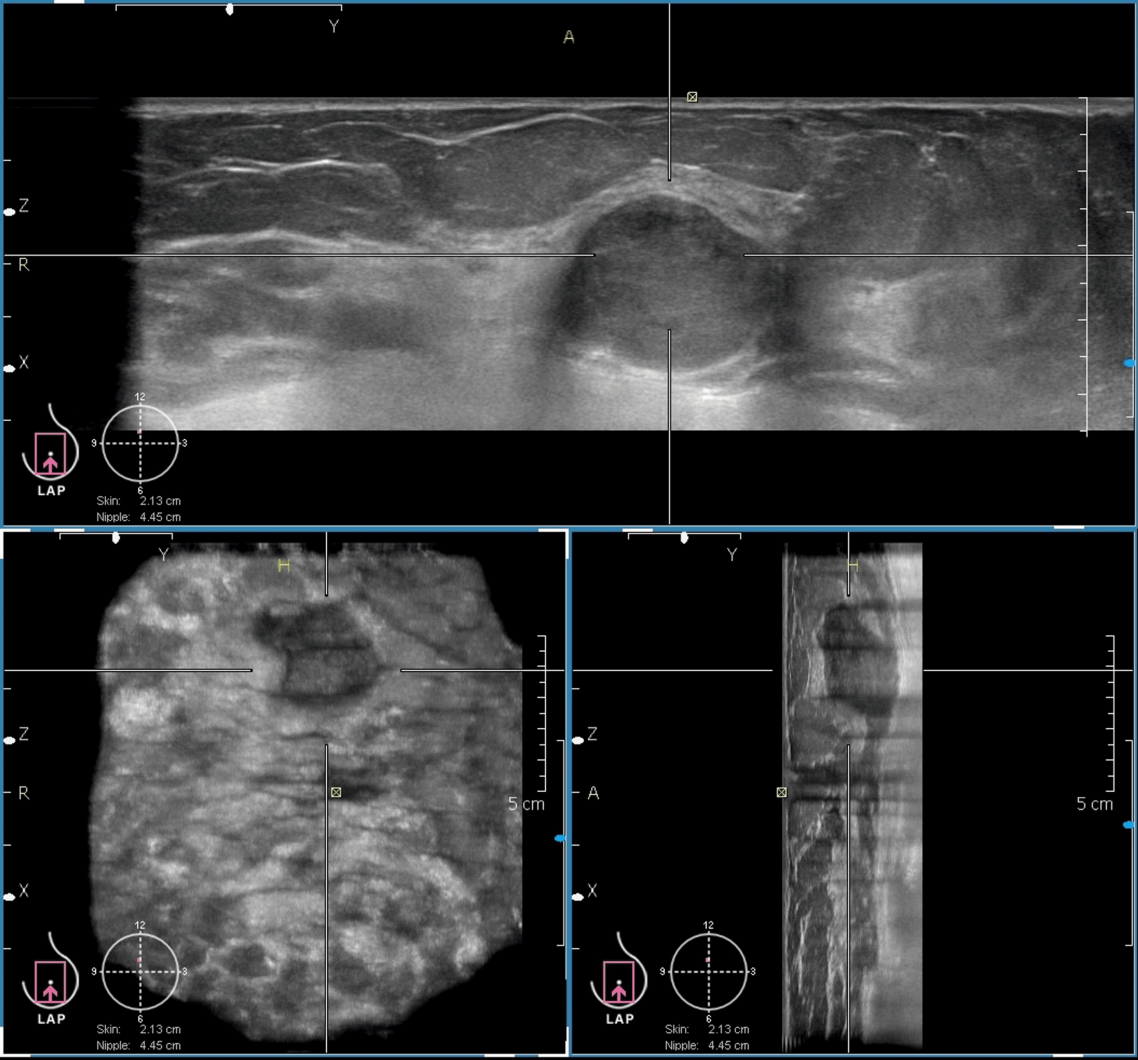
ABVS image of the 30-year-old patient The image is demonstrating a lesion at the 12 o'clock position of the left breast, consistent with a fibroadenoma. The lesion exhibits imaging characteristics similar to those observed on HHUS, highlighting the concordance between both modalities in lesion assessment. ABVS: Automated breast volume scan, HHUS: Hand-held ultrasound.
